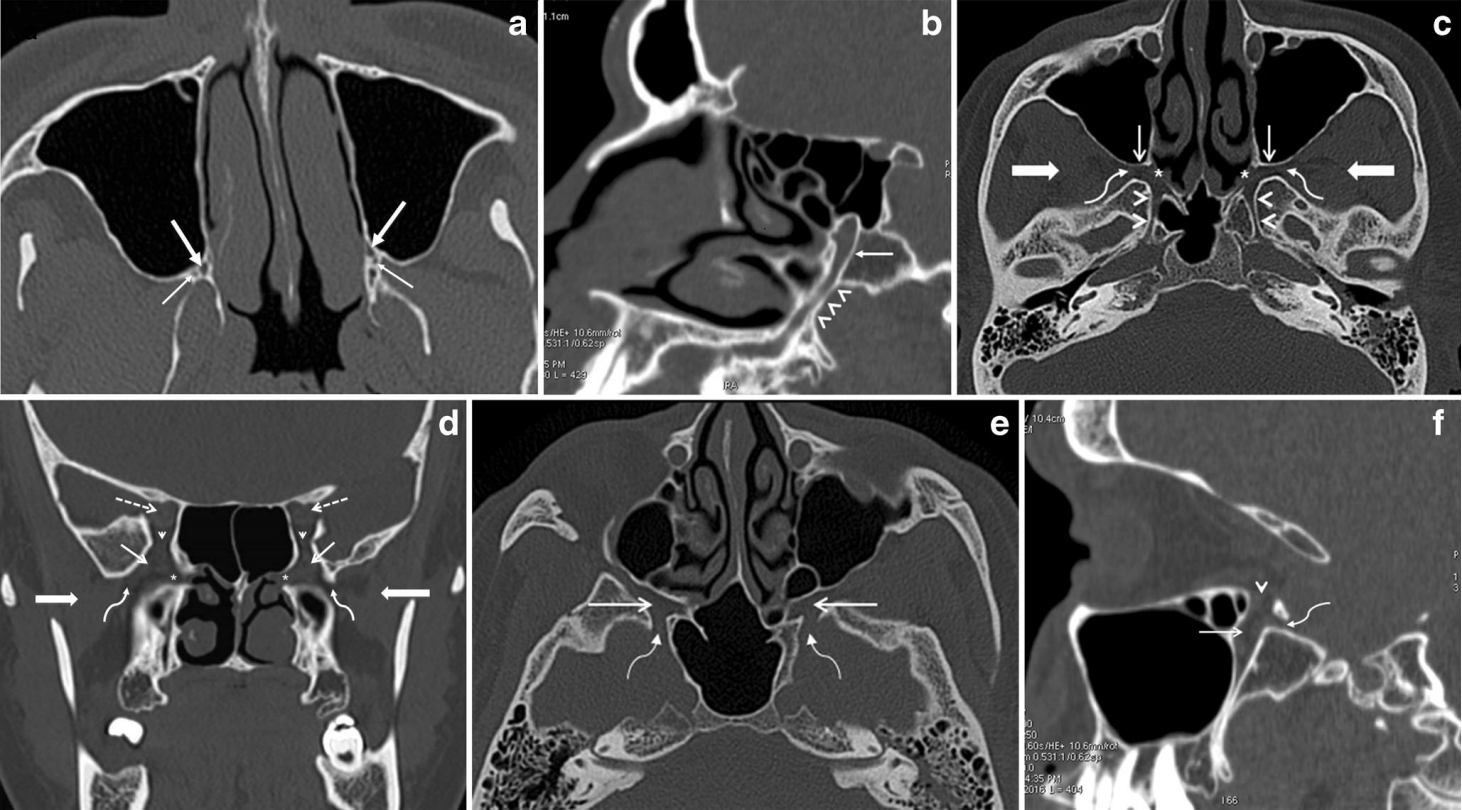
a Axial HRCT image shows the greater (*thick arrows*) and lesser (*thin arrows*) palatine canals at the inferior apex of the PPF. b Oblique HRCT reconstruction through the greater palatine canal (*arrowheads*) shows its entire longitudinal course, from the hard palate inferiorly to the apex of the PPF superiorly (*arrow*). c Axial HRCT image through the PPF obtained at the level of the Vidian canal. The PPF (*thin straight arrows*) communicates medially with the nasal cavity via the SPF (*asterisk*) and laterally with the ITF (*thick straight arrows*) via the PMF (*curved arrows*). The Vidian canal (*arrowheads*) extends from the PPF to the foramen lacerum on each side. d Coronal HRCT image through the PPF at the level of the IOF. The PPF (*thin straight arrows*) communicates with the ITF (*thick straight arrows*) via the PMF (*curved arrows*). The SPF (*asterisk*) opens into the nasal cavity medially and the IOF (*arrowheads*) opens into the orbital apex (*dashed arrows*). e Axial HRCT image through the PPF obtained at the level of the foramen rotundum. The PPF (*straight arrows*) communicates with the middle cranial fossa via the foramen rotundum (*curved arrow*) on each side. f Sagittal HRCT reconstruction through the PPF (*straight arrow*) showing its communication with the orbit via the IOF (*arrowhead*) and with the middle cranial fossa via the foramen rotundum (*curved arrow*) at the same level
